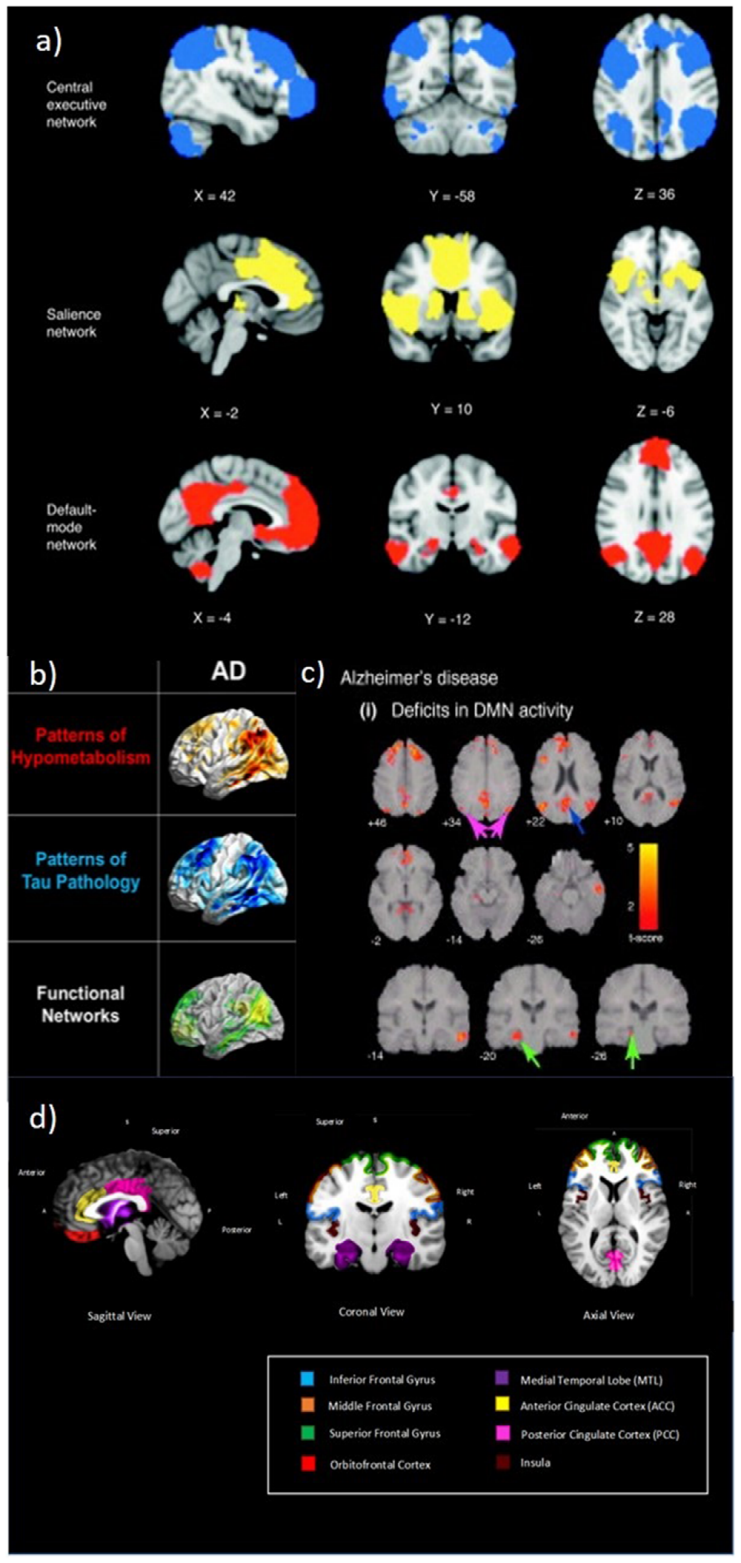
A, Principal brain regions in the central executive, salience, and default‐mode networks (adapted from Menon.^78^ B, Overlap between hypometabolism on 18F‐FDG PET, tau aggregation on 18F‐T807 PET, and default mode networks from resting‐state functional MRI in AD (adapted from Drzezga^79^). C,  Changes in DMN activity in patients with AD compared to age‐matched healthy elderly controls. The PCC (blue arrow), angular gyrus in the inferior parietal cortex (magenta arrow) and hippocampus (green arrow) show prominent activity changes in AD (adapted from Menon^78^). D, Common regions of atrophy and functional impairment associated with deficits in higher level awareness and metacognition in early AD (adapted from Hallam and Huntley^73^). AD, Alzheimer's disease; DMN, default mode network; FDG, fluorodeoxyglucose; MRI, magnetic resonance imaging; PCC, posterior cingulate cortex; PET, positron emission tomography
